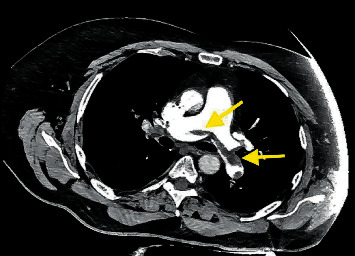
Computed tomography angiography (CTA) of the chest showing bilateral pulmonary embolism (yellow arrows).
